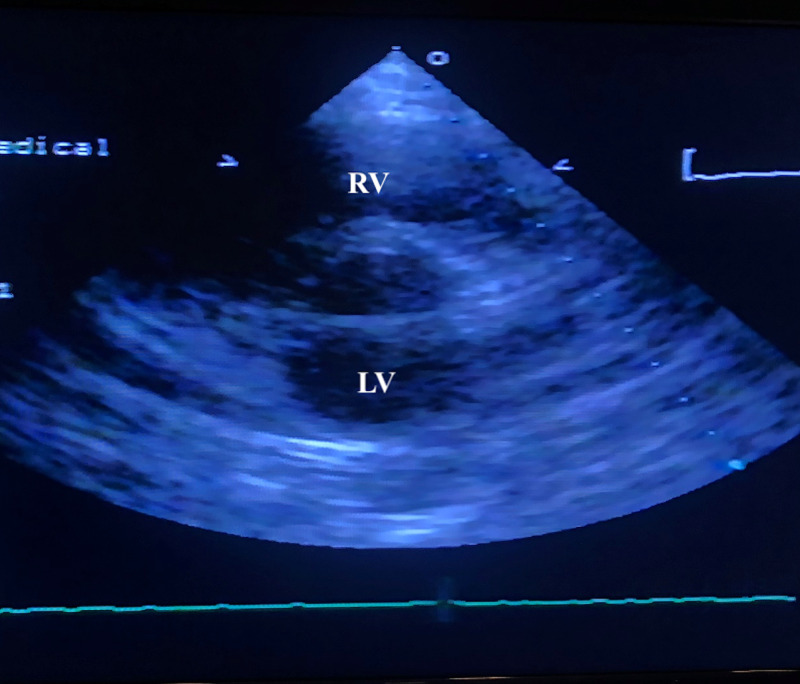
Echocardiogram Parasternal short-axis view showing normal right ventricle and left ventricle
